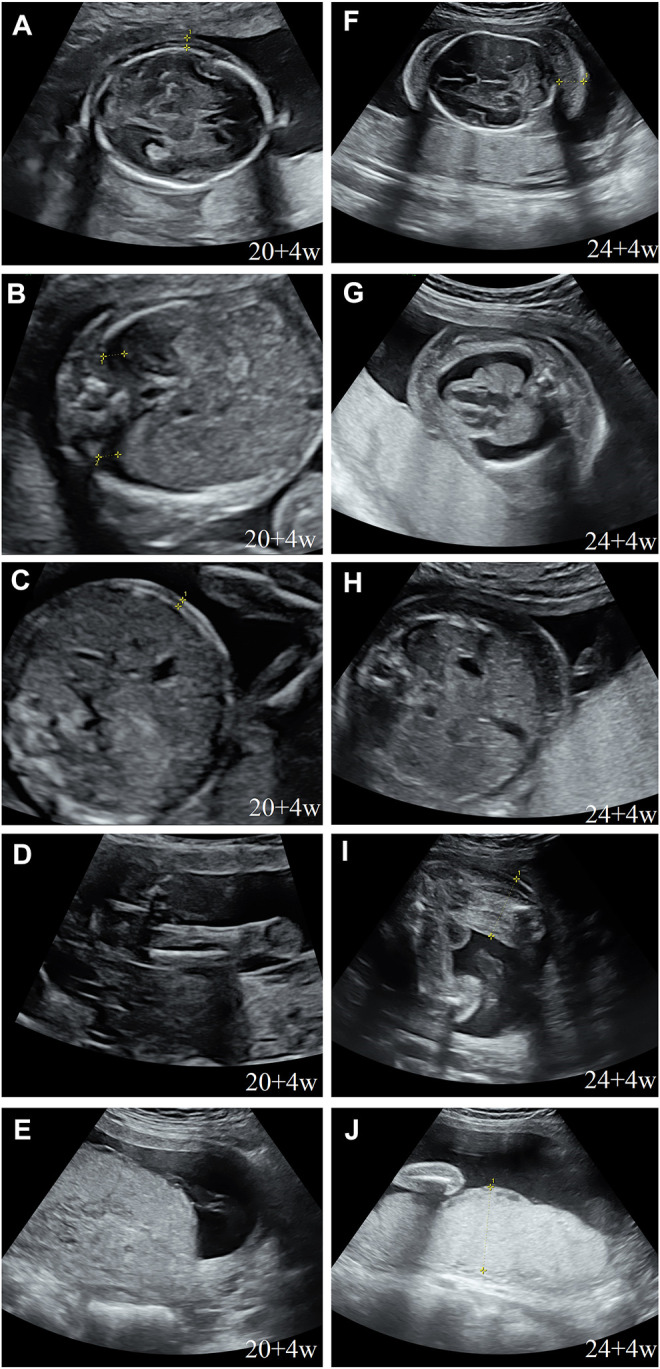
Prenatal ultrasound. (A) Thalamus transverse section: subcutaneous edema: 3 mm; (B) thorax transverse section: bilateral hydrothorax: 4 mm; (C) abdominal transverse section: no subcutaneous edema; (D) sagittal section of the thigh: no subcutaneous edema; (E) no placental thickening; (F) cerebellum transverse section: subcutaneous edema: 14 mm; (G) thorax transverse section: subcutaneous edema: 10 mm, bilateral hydrothorax: 10 mm, and lung collapse, heart pressed; (H) abdominal transverse section: subcutaneous edema: 10 mm; (I) sagittal section of the thigh: subcutaneous thickening: 9 mm; and (J) placental thickening: 53 mm.
